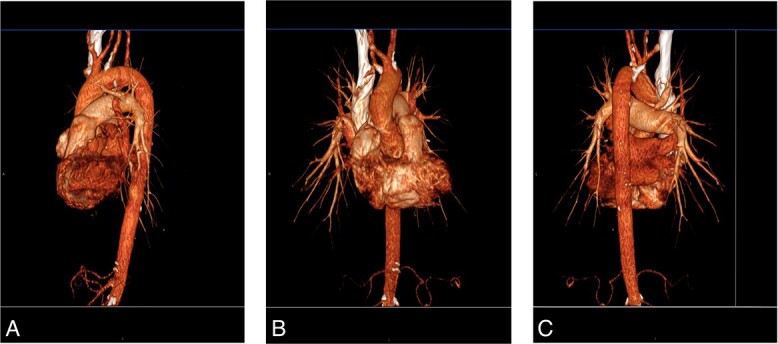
(A) CTA, left lateral view. (B) CTA, anterior view. (C) CTA, left posterior oblique view.
